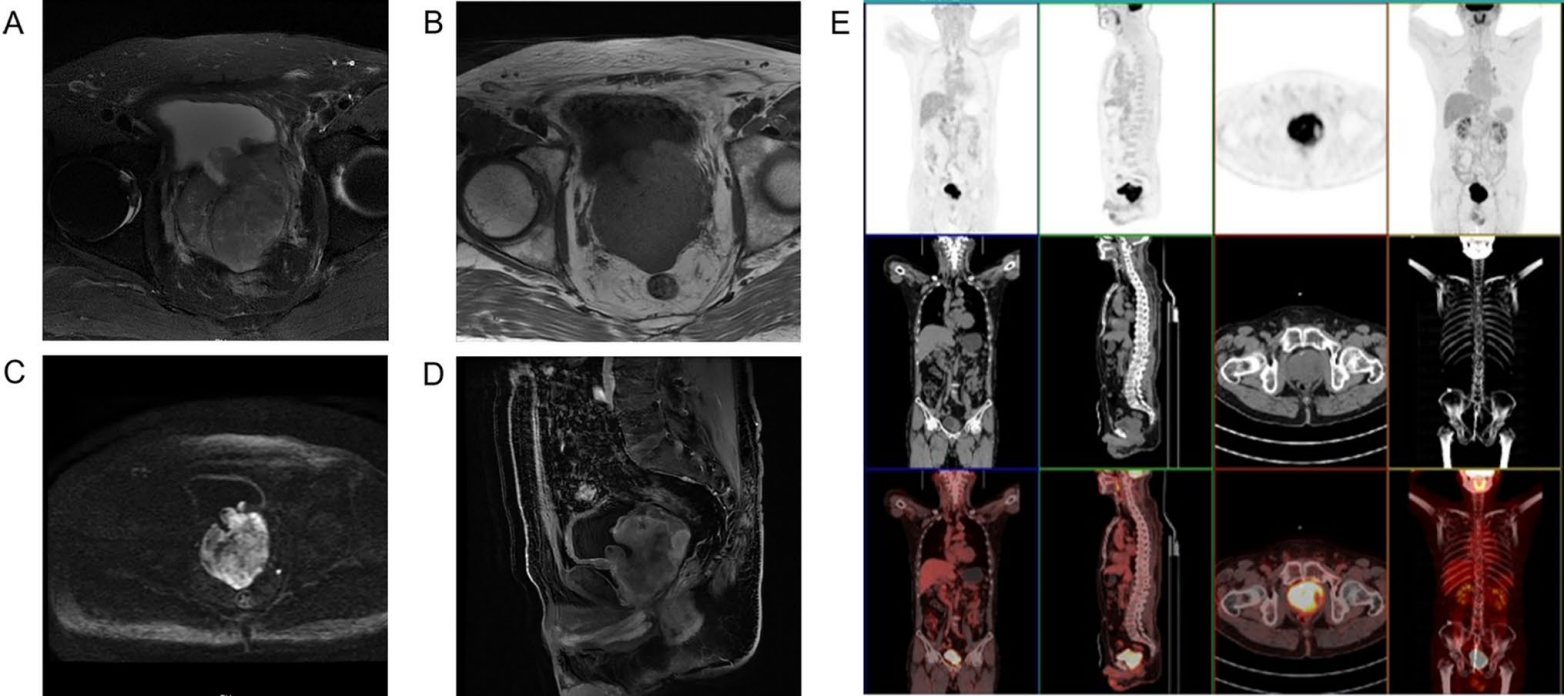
MR showed irregular tumor mass in prostate with uneven signal on T2WI image, obviously high signal on DWI image. A T1WI image, B T2WI image, C DWI image, D water image (sagittal). E PET–CT showed abnormal high glucose metabolism of the prostate but no distant organ metastasis was found
